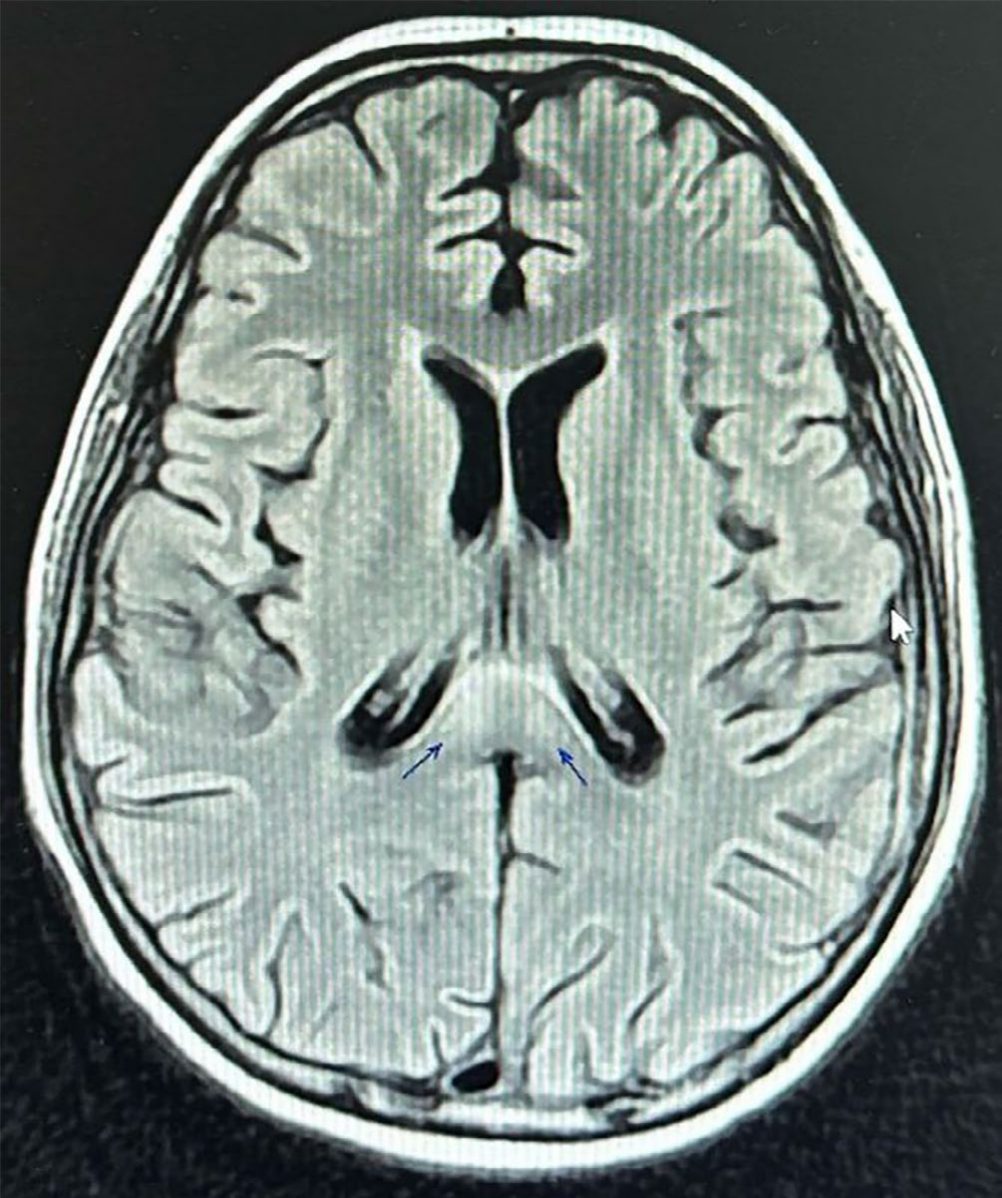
Brain MRI. The image shows two foci of altered signal intensity within the splenium of the corpus callosum (pointed with arrows) eliciting bright T2/FLAIR signal intensity with mild restriction in the DWIs, suggestive of cytotoxic lesions.
